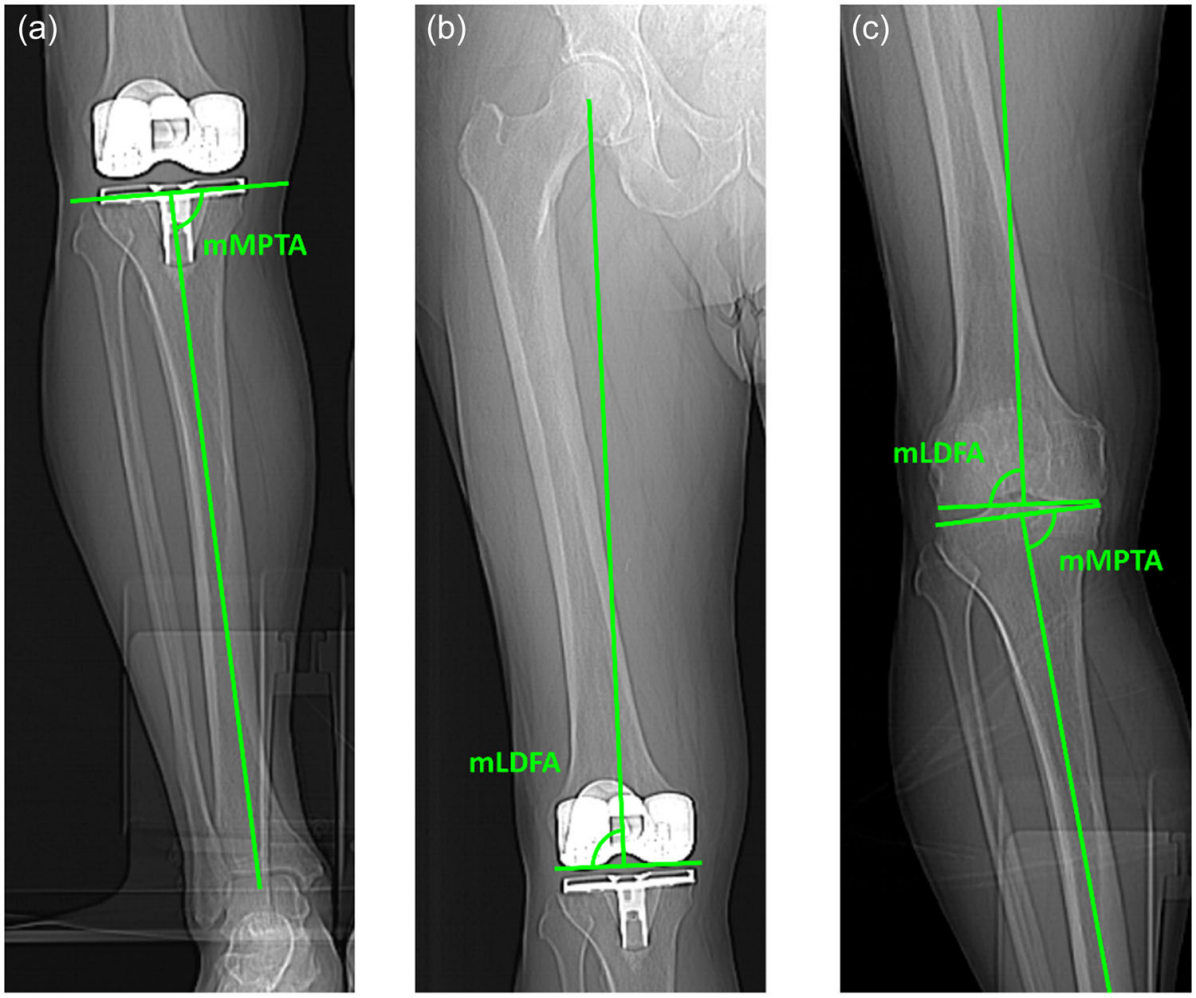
CT femoral and tibial alignment measurement in the coronal plane. CT, computed tomography; mLDFA, mechanical lateral distal femoral angle; mMPTA, mechanical medial proximal tibial angle.
